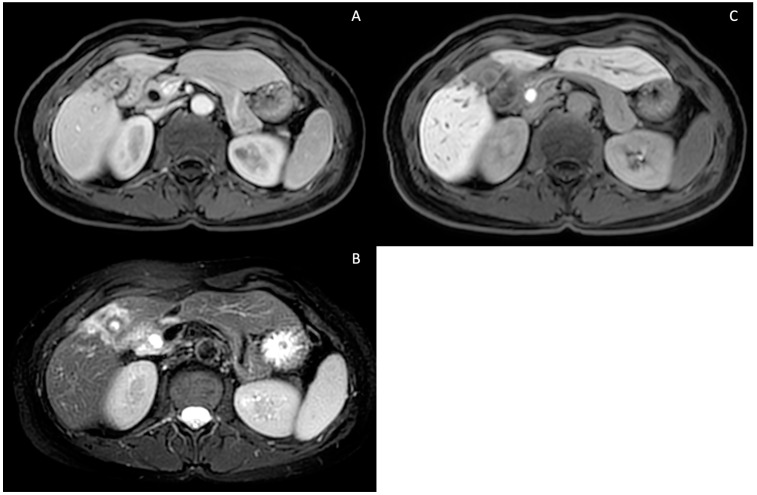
Intrahepatic pericholecystic cholangiocarcinoma. T1-weighted contrast-enhanced portal phase (A), T2-weighted sequence showing hyperintensity due to increased cellularity (B) and T1-weighted contrast-enhanced (Gd-EOB-DTPA) hepatospecific phase showing hypointensity due to a lack of hepatocellular contrast uptake (C).
